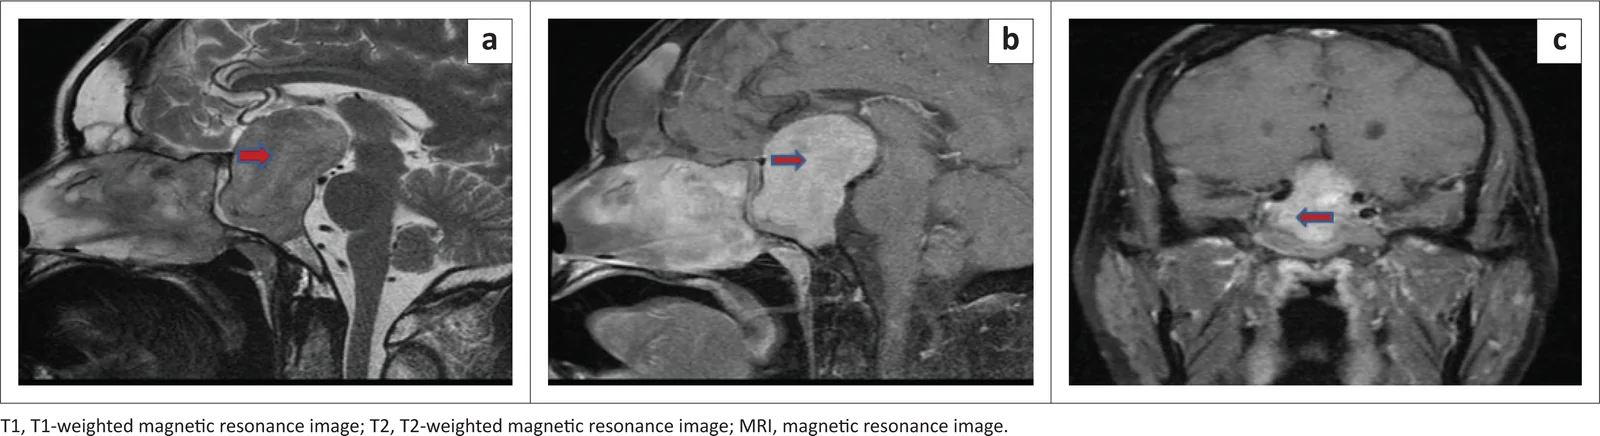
Brain magnetic resonance images of one of the invasive pituitary neuroendocrine tumours (giant adenoma) seen in the study, Department of Anatomic and Molecular Pathology, Lagos University Teaching Hospital, Lagos, Nigeria, 10 June 2019 – 10 June 2020: (a) Sagittal T2-weighted brain MRI without contrast showing a giant suprasellar mass (arrow) with hypointense marrow changes in the clivus suggesting infiltration (Hardy’s grade III); (b) Sagittal T1-weighted brain MRI with contrast showing a hyperintense sellar mass (arrow) with suprasellar extension, the mass has eroded the floor of the sella turcica as well as the upper clivus and it compresses the optic chiasma displacing it superiorly (Hardy’s grade III); (c) Coronal T1-weighted brain MRI with contrast showing an invasive suprasellar mass (arrow) with infiltration of the cavemous sinus as well as partial encasement of the intemal carotid arteries on both sides (Knosp’s grade III).
